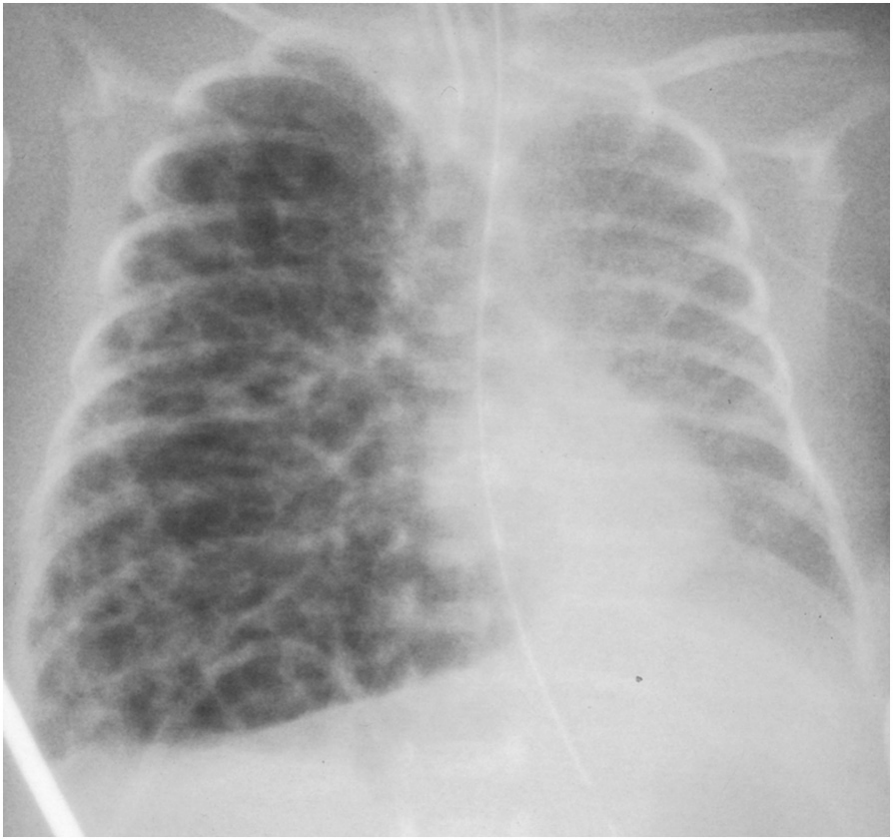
A chest X-ray obtained on postnatal day 11. Diffuse pulmonary interstitial emphysema with growing pseudocystic changes in the right lung field can be seen on this X-ray.
